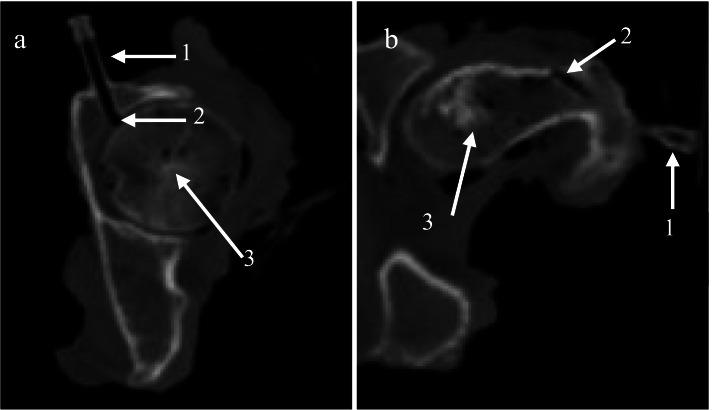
a, b Axial (superior) view using computed tomography of the intra-osseous tunnels. Small arrows show the injector chamber (1), intra-osseous tunnel entrance (2) and the femoral head (3)
